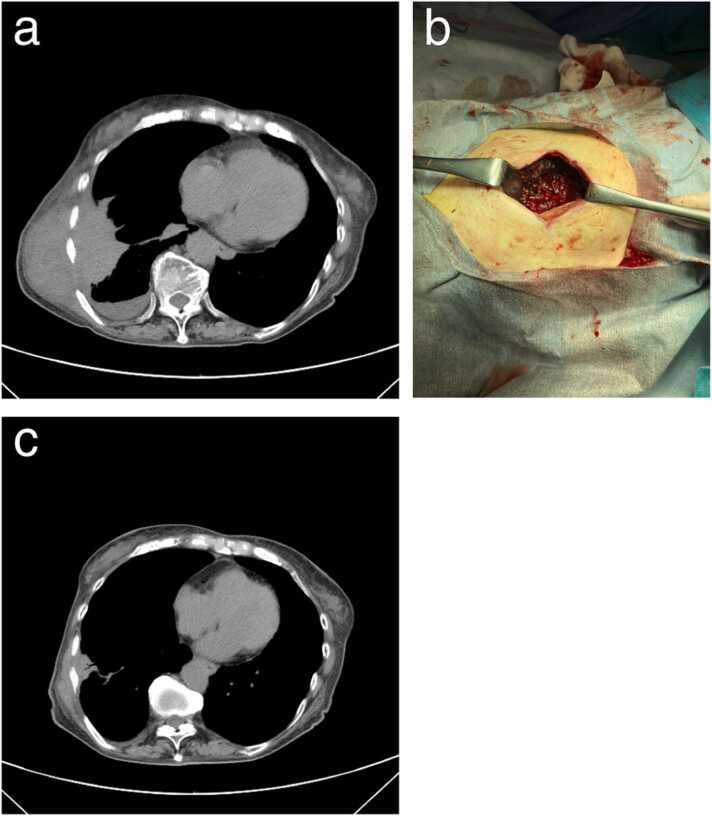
Clinical findings during the second admission of the patient. (a) Plain CT scan of the chest revealed a relapse of empyema necessitans, characterized by increases in subpleural lung abscess, pleural effusion, and subcutaneous abscess. (b) A photograph of the surgical incision into the subcutaneous mass taken on day 4 of the second admission. (c) A plain chest CT scan performed after treatment with antibiotics and open drainage with debridement showed marked improvement of lung abscess, pleural effusion, and subcutaneous abscess.
